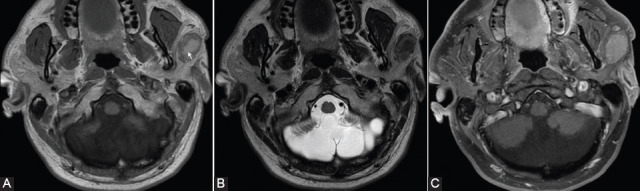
Conventional magnetic resonance imaging appearance in a 50-year-old patient with a Warthin tumor of the left parotid gland. (A) Non-enhanced T1-weighted image (WI) sequence, with inhomogeneous aspect, with central hyperintense area with hemorrhagic transformation (arrow). (B) T2-WI sequence showing a hypointense central area. (C) T1 contrast-enhanced sequences showing enhancement, slightly inhomogeneous aspect.
